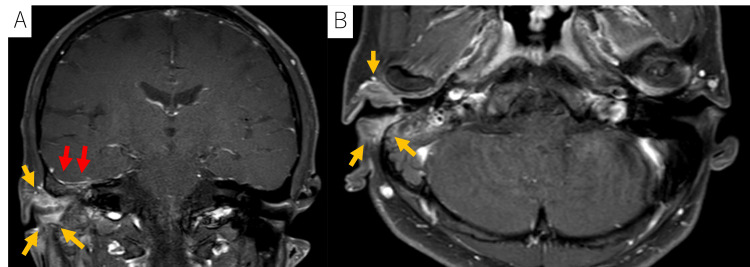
Images of the tumor in case 2 A, B: MRI images revealing the tumor (indicated by yellow arrows) and enhancement of the dura mater in the right middle cranial fossa (indicated by red arrows). The disease was classified as T4 based on the modified Pittsburgh classification. The patient had no cervical lymph node involvement or distant metastasis.
